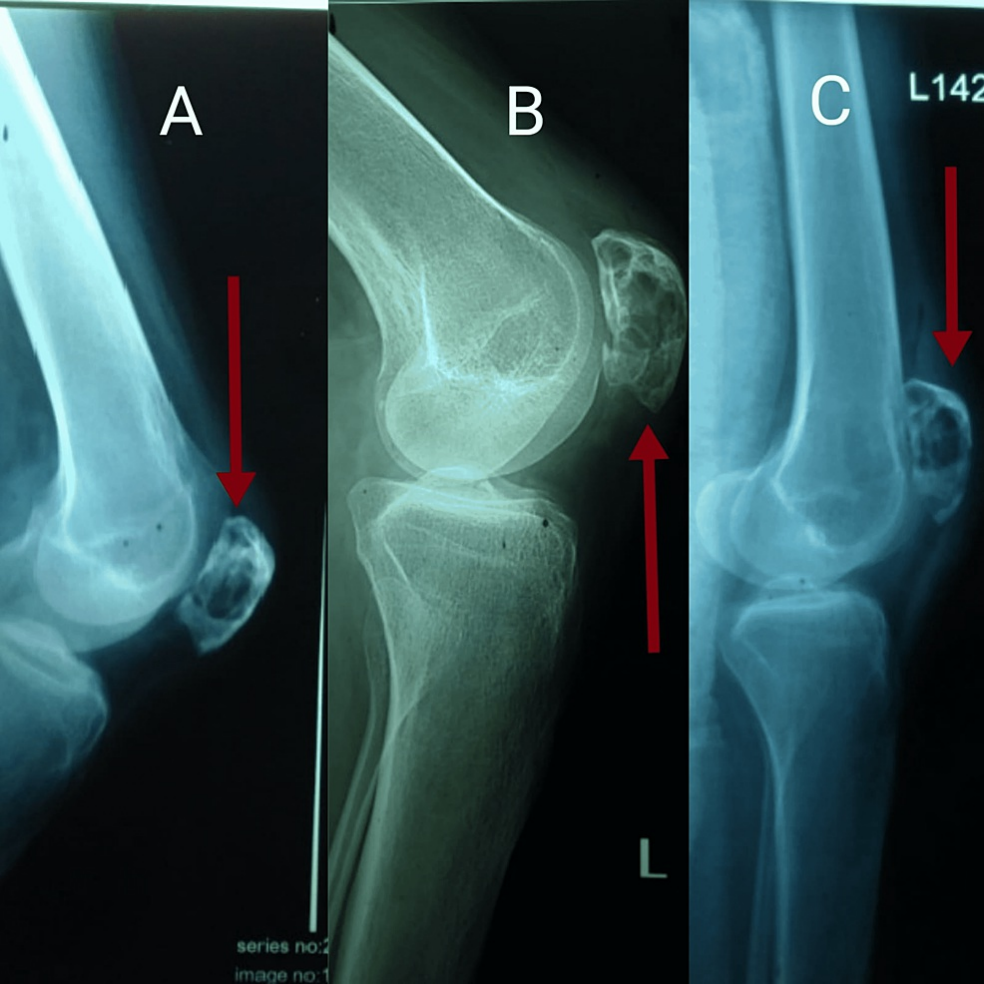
Lateral radiographs of the left knee joint (A-C): well-defined lytic lesions throughout the substance of patellar bone.
